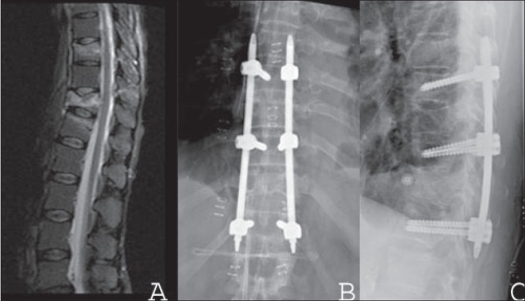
Mid sagittal T2WI (A) MRI shows flexion-distraction injury of the thoracolumbar spine in a 55 yrs old female with no neural deficit. X-ray of dorsal spine AP and lateral (B,C) shows stabilization with percutaneous longitude Longitude™ implant. Early ambulation without external bracing was achieved on day one post surgery. Wound healing was primary; no substantial complaints were reported on follow-up.
